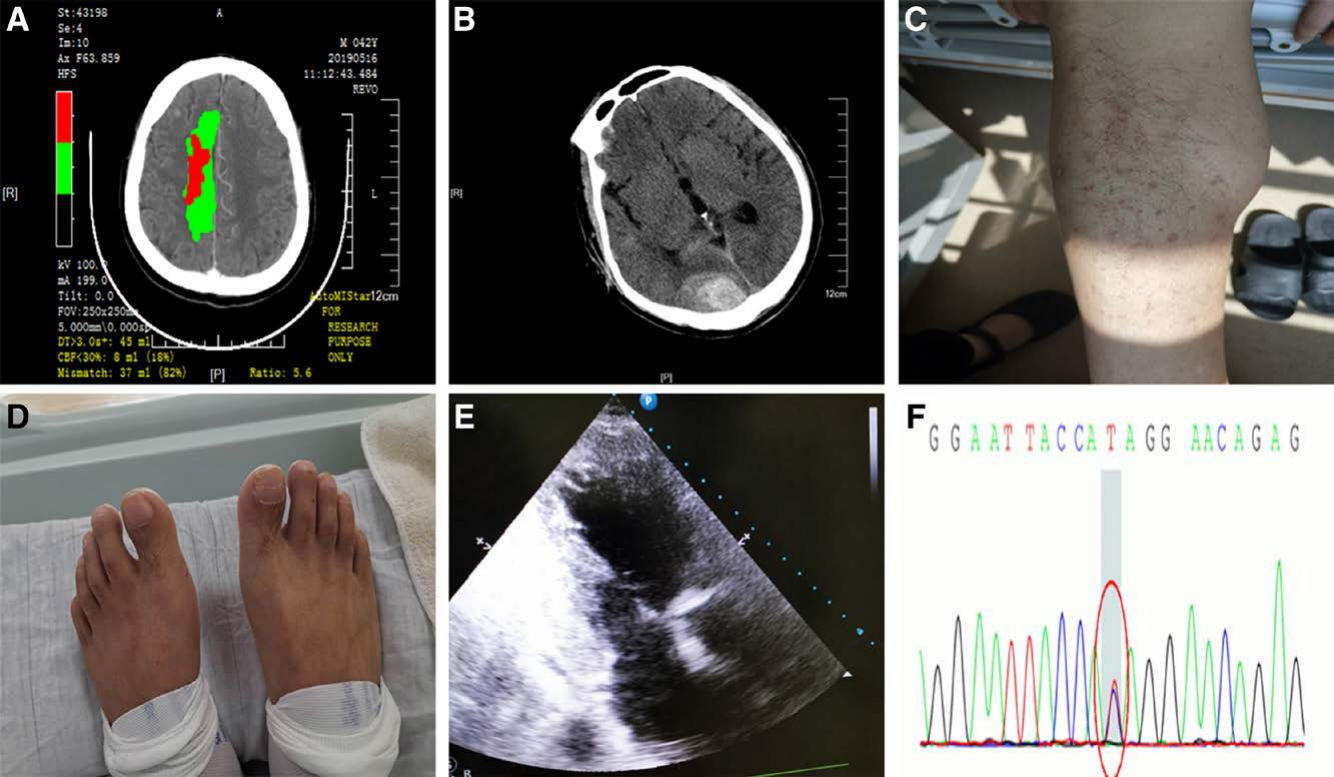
Radiologic findings, physical examination, and gene test results of the patient. (A) CTP showed ischemic perfusion in the blood supply territory of the A4 segment of the right anterior cerebral artery, with a volume mismatch of 82%. (B) Axial cerebral CT scan image reveals hyperdensities in the right occipital lobe along with subarachnoid hemorrhage subsequent to intravenous thrombolysis (IVT). (C) Skin lesions were painless hemorrhagic erythema on both lower limbs. (D) Clubbing of toes. (E) Echocardiogram revealed vegetations in the mitral valve. (F) NGS results for the patient and his mother; both had a heterozygous mutation of c.1177A > G on chr15-48808530. CT = computed tomography, CTP = CT perfusion.
